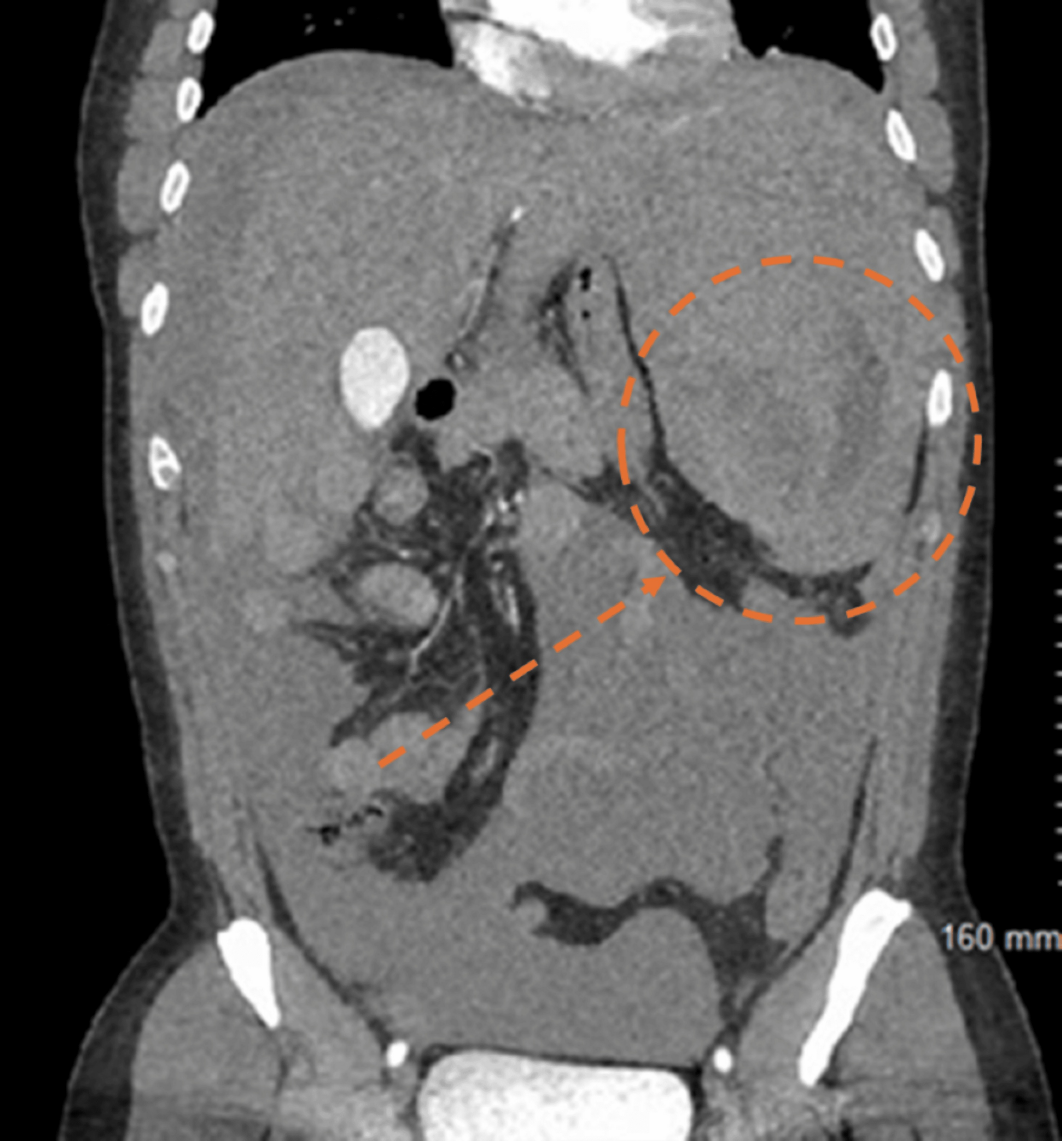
Coronal CT scan of the abdomen and pelvis. Demonstrates a splenic rupture with associated findings, including evidence of intra-abdominal hemorrhage and splenic parenchymal disruption.
